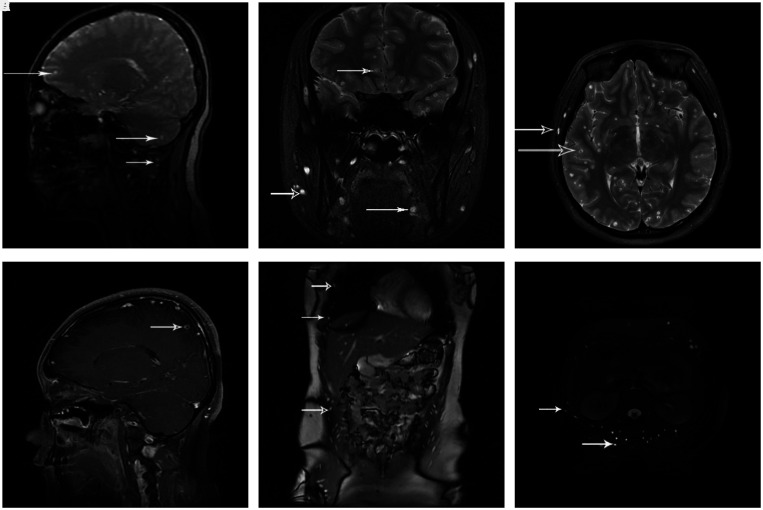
(A, B, C, and D) Magnetic resonance imaging of the head (March 2018) reveals multiple cystic lesions in the bilateral cerebral cortex, parenchyma and cerebellum, subcutaneous tissues, and muscles of the face, neck, and tongue. (E and F) Magnetic resonance imaging of the abdomen (March 2018) reveals cystic lesions in the abdominal muscles, liver, peritoneum, and erector spinae muscle.
